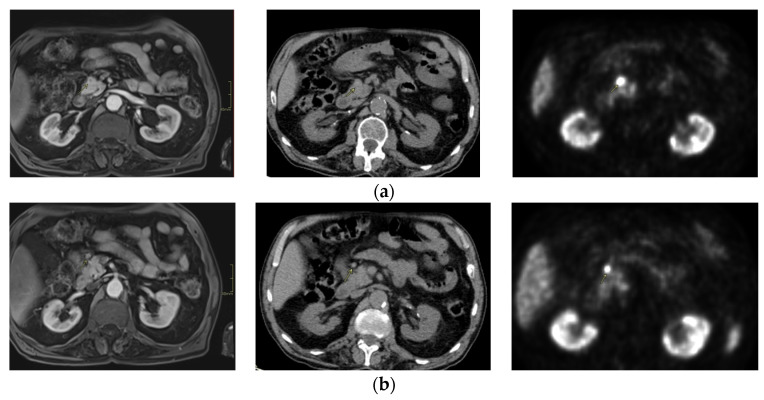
(a) T1 vibe Dixon MRI, CT, and PET CT of the abdomen performed in October 2018. The tumor on the abdominal part of the head of the pancreas. (b) T1 vibe Dixon MRI, CT, and PET CT of the abdomen performed in October 2018. Peripancreatic lymph node.
